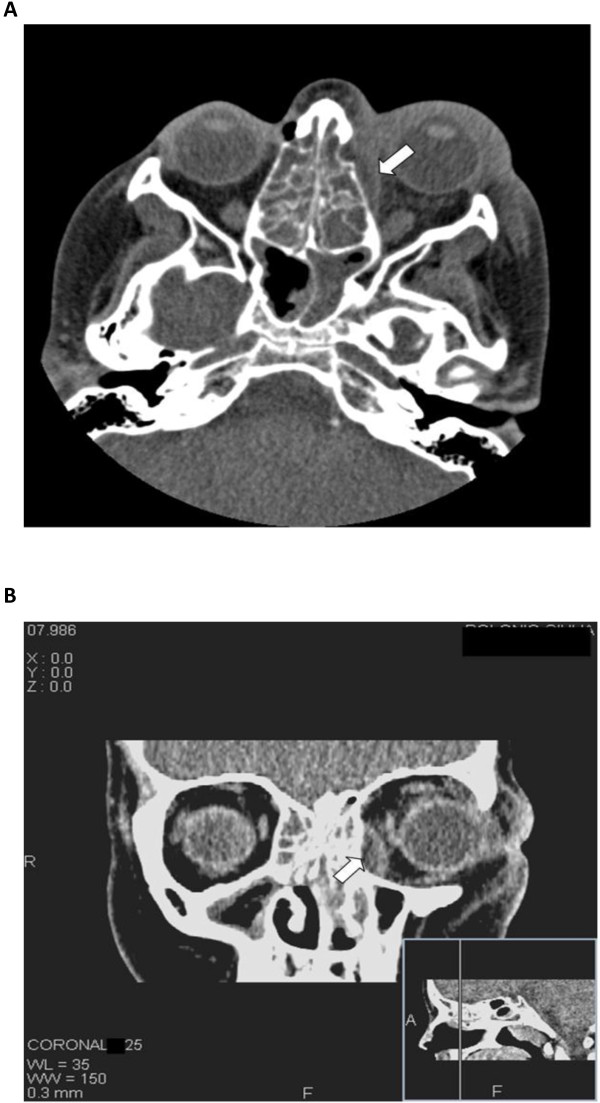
A-B. Axial (A) and coronal (B) CT unenhanced scan through the middle orbit. The images show: increased thickness of skin and soft tissue overlying the medio-lateral area of the left orbit, decreased transparency of the ipsilateral intraconal and extraconal fat tissue and a subperiosteal abscess along the medial wall of the left orbit (white arrows), adjacent to the opacified ethmoid air cells with resultant lateral displacement of the medial rectus muscle (b) A partial obliteration of sphenoid (A) and maxillary sinuses (B) is also visualized.
